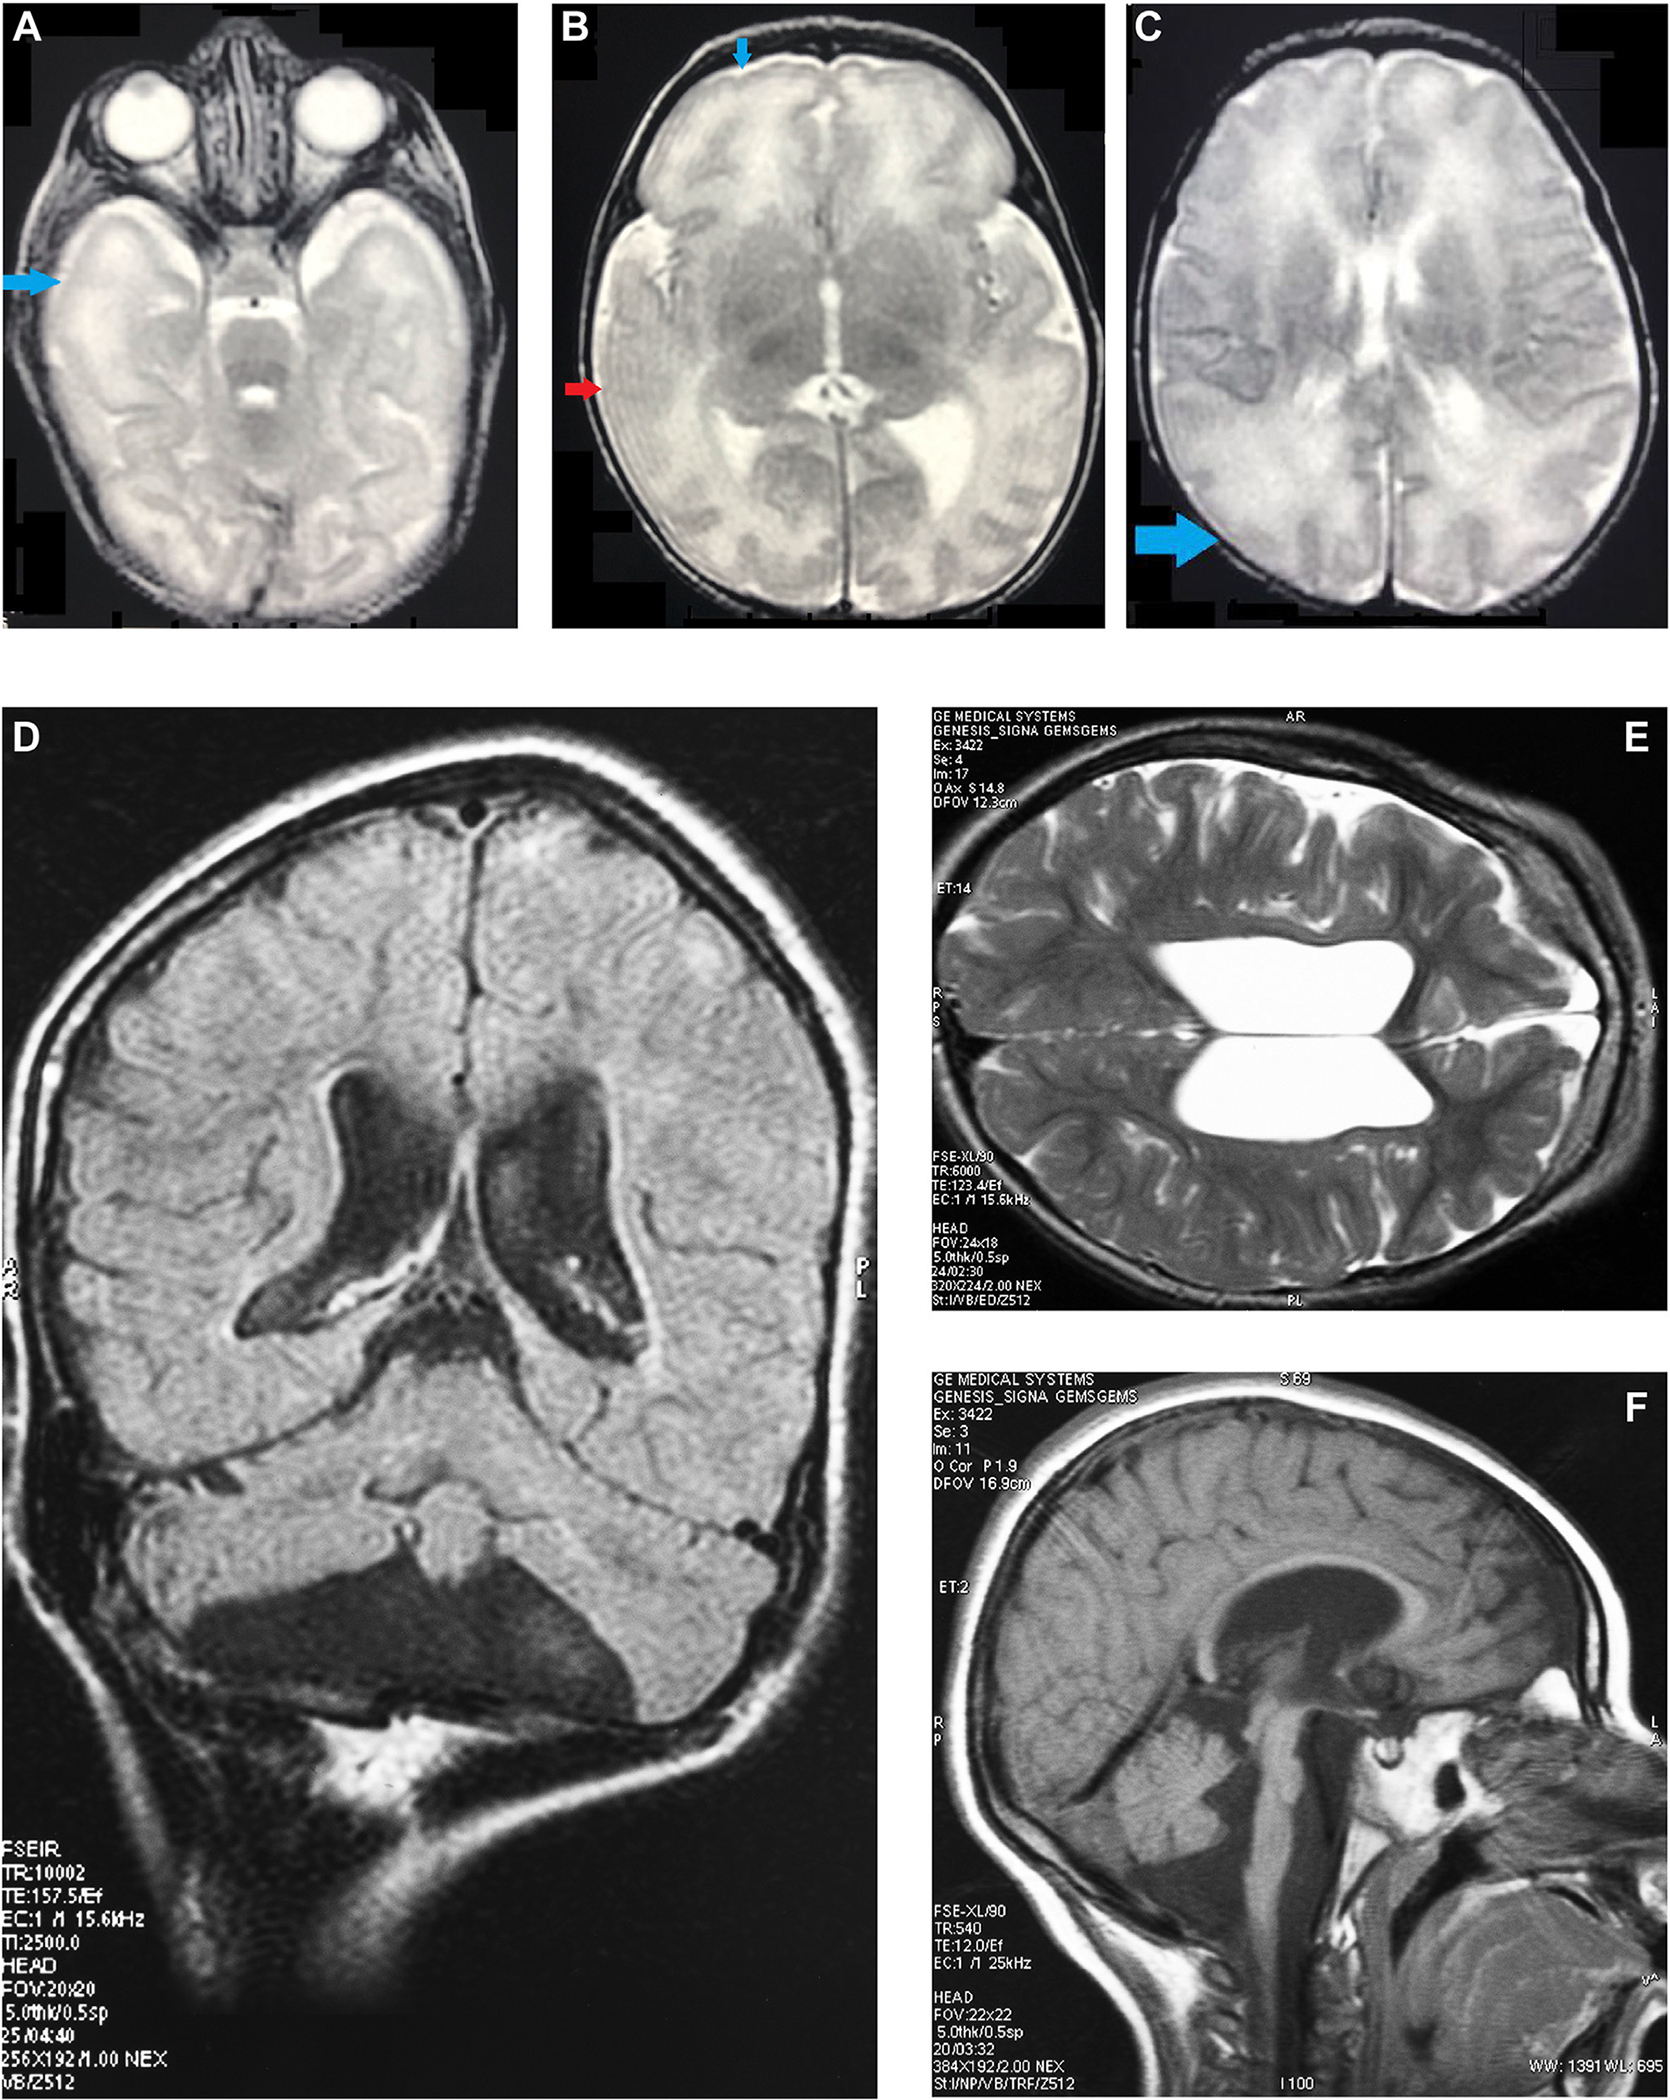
Magnetic Resonance Images (MRI) of patient 1 and 3. T2-weighted images at the age of 5 days of patient 1 showed regions in both hemispheres with a simplified gyral pattern, indicated by blue arrows in (A), (B), and (C). The red arrow in (B) marks a possible abnormal lateral right temporal lobe, but the actual anatomy is obscured by a Gibbs artifact. MRI of patient 3 at the age of 4 years ([D] (FLAIR), [E] (T2 weighted), and [F] (T1 weighted) showed enlarged lateral and third ventricles, decreased volume of cerebral white matter and incomplete myelination, and pontocerebellar atrophy.
